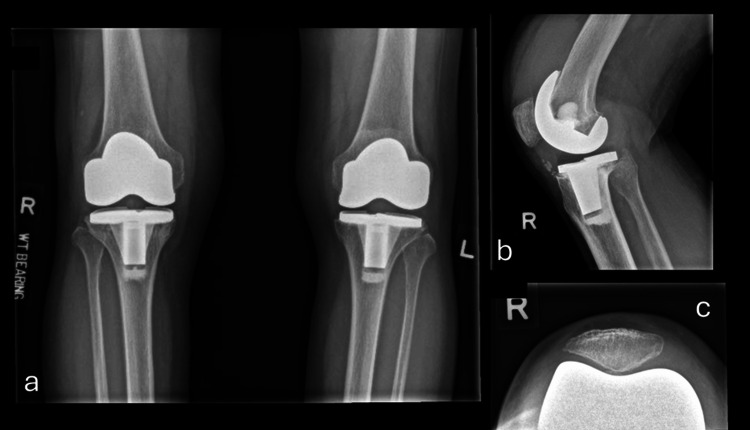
Knee radiograph during initial presentation. (a) Anteroposterior view bilateral knee; (b) Lateral view right knee; (c) Skyline view right knee.
